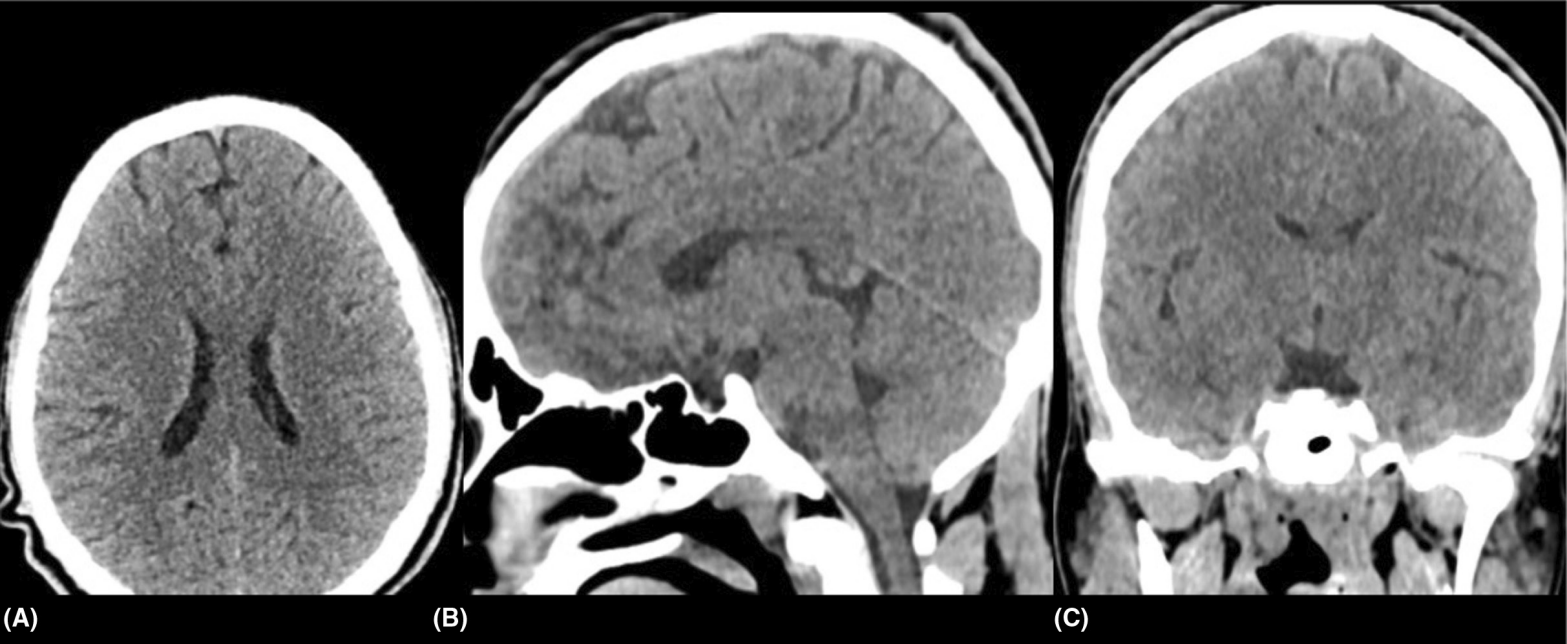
Non‐contrast CT head: (A) axial, (B) sagittal and (C) coronal cuts, showed no acute pathology.
